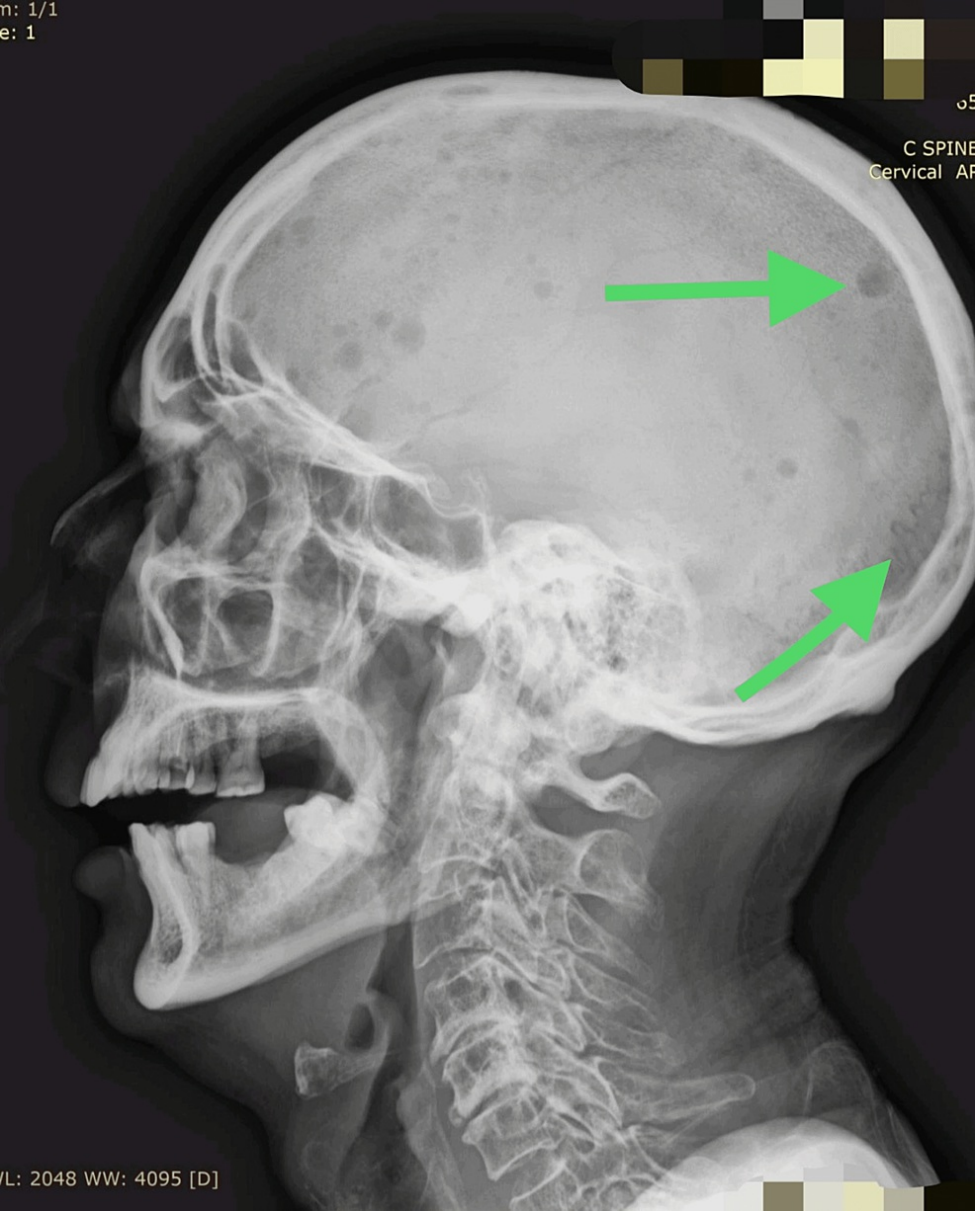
An X-ray of the skull (lateral view) with the arrows indicating lytic lesions in the skull
